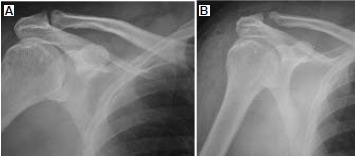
Fig. 5: MRI right shoulder sagittal view showing marked arthropathic changes and the axial view with oedema below the acromioclavicular joint with supraspinatus impingement. F5:
